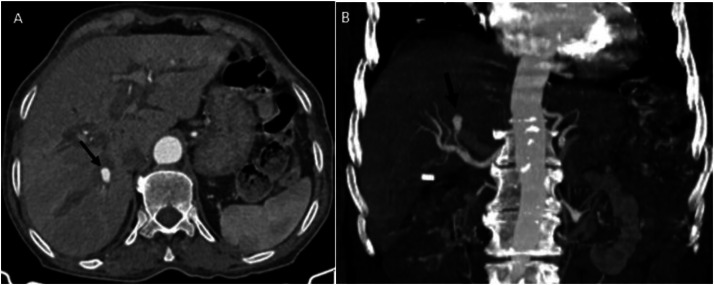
Contrast enhanced computed tomography scan showing an enhancing cavity in segment VII communicating with a sectoral branch of the hepatic artery (black arrow). (A and B).
